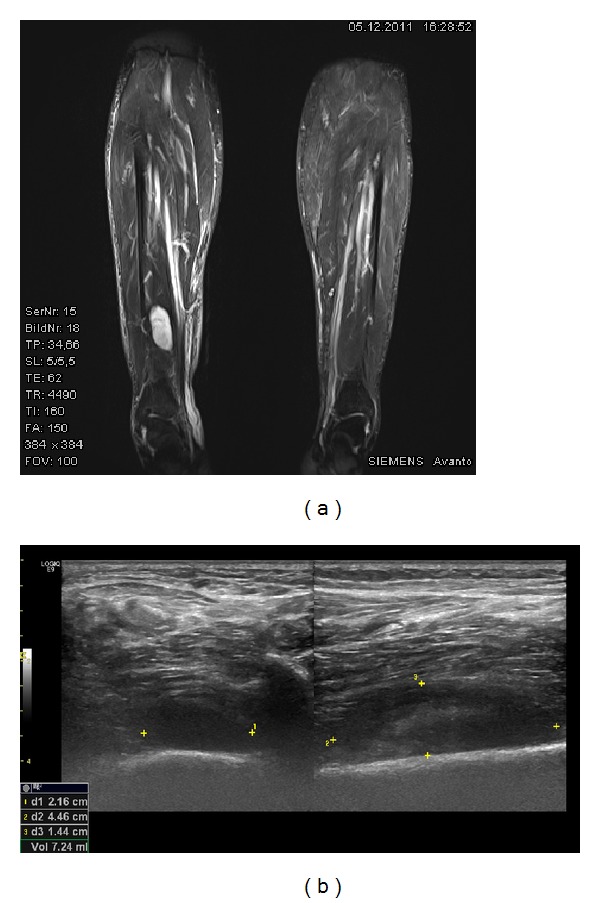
(a) MRI of the giant cell tumor at the distal tibia and (b) an ultrasound examination of the tumor.
